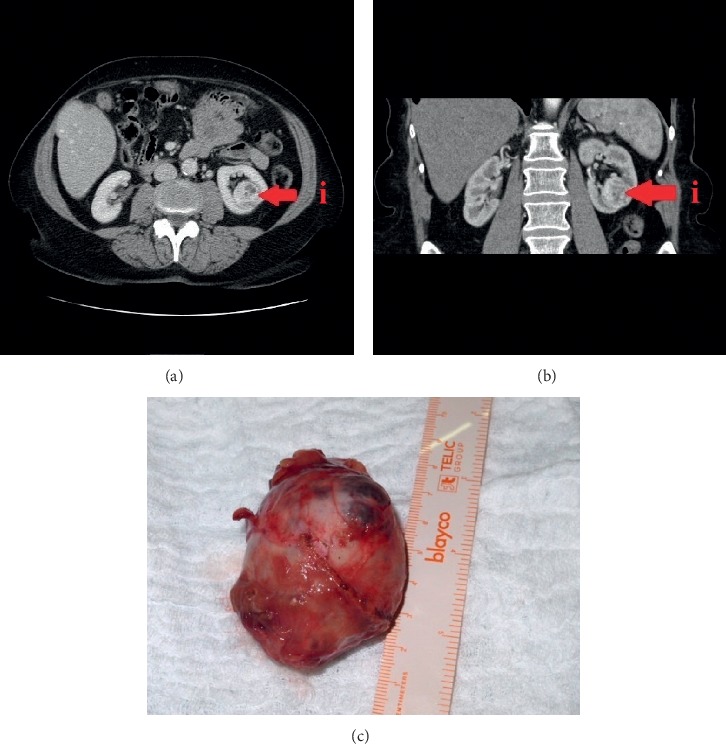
(a) Preoperative CT imaging of (i) endophytic renal tumor before partial nephrectomy in axial projection. (b) Preoperative CT imaging of (i) endophytic renal tumor before partial nephrectomy in coronary projection. (c) Excised renal tumor after partial nephrectomy.
